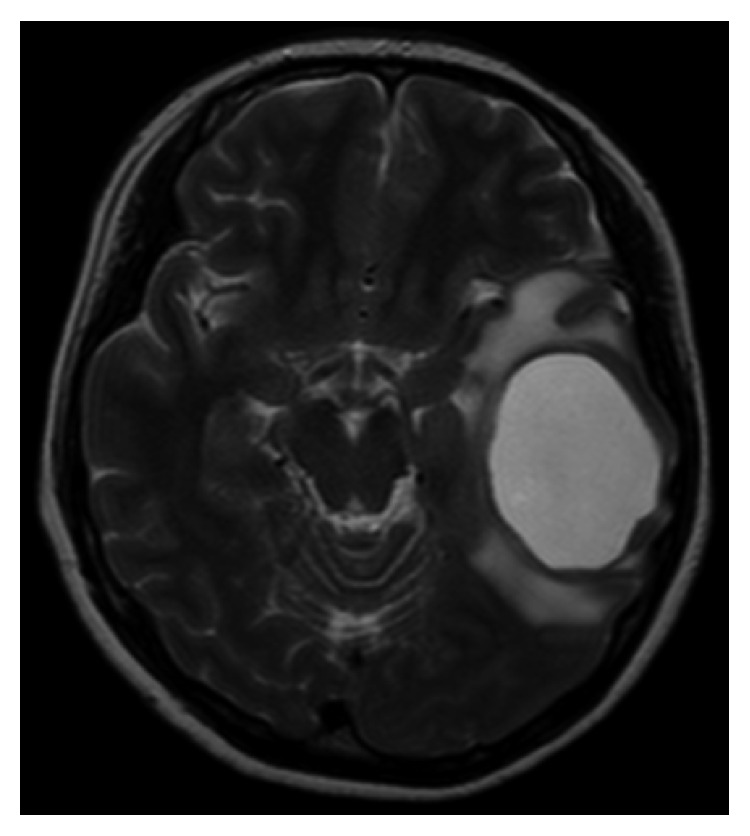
Brain magnetic resonance imaging (horizontal) indicating a solid mass in the left temporal lobe.
